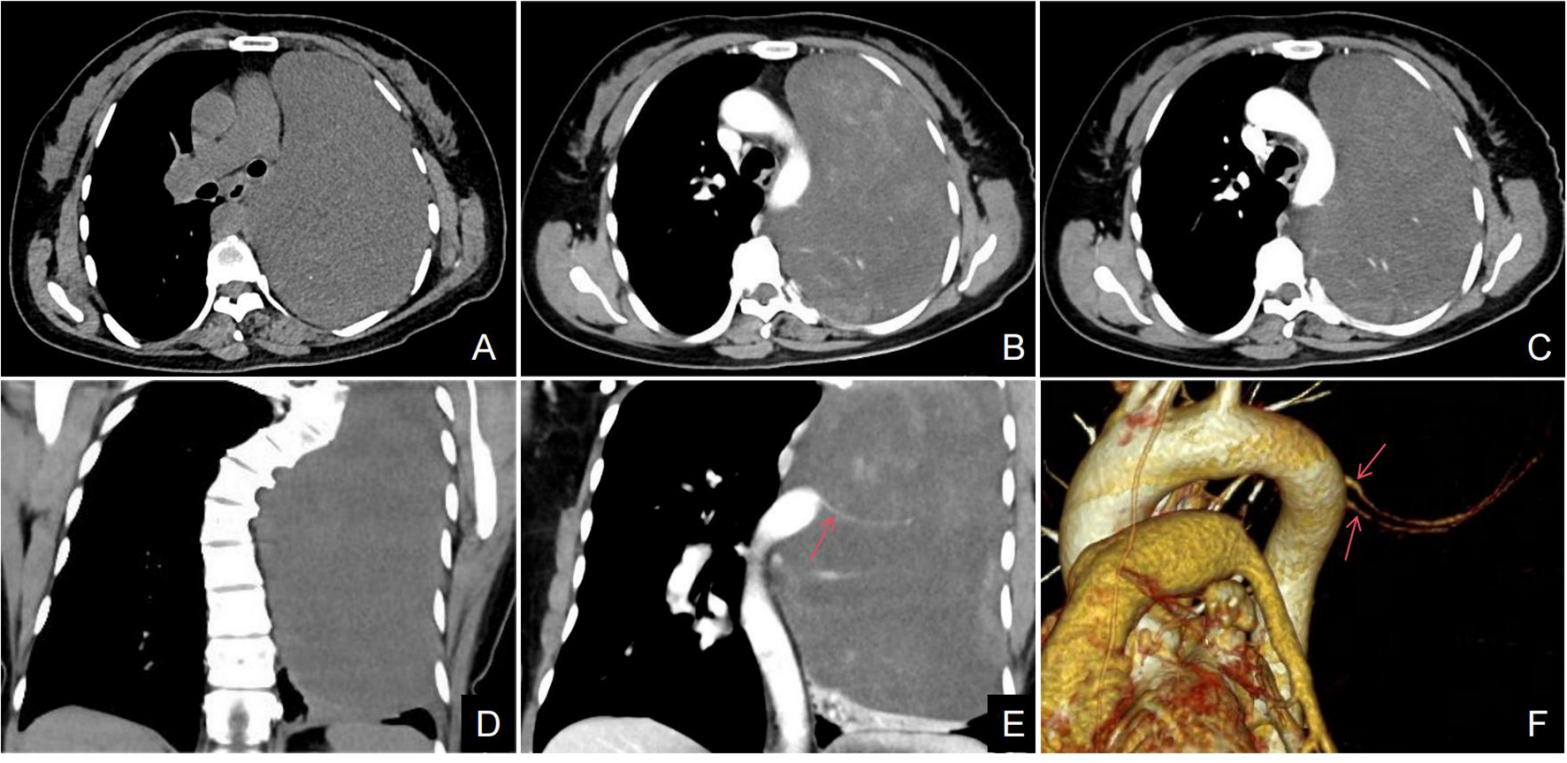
(A–F) Axial CT scan, a large well-defined mass with punctate calcifications was seen in the thoracic cavity (A). The axial CT enhancement image shows uneven delayed enhancement of the mass with a “patchy” appearance (B, C); the arterial phase of the coronary CT and VR (red arrow) clearly shows that the trophoblastic vessels of the mass are from the abdominal aorta (E, F). Coronal CT scan showed scoliosis in relation to the tumor (D).
